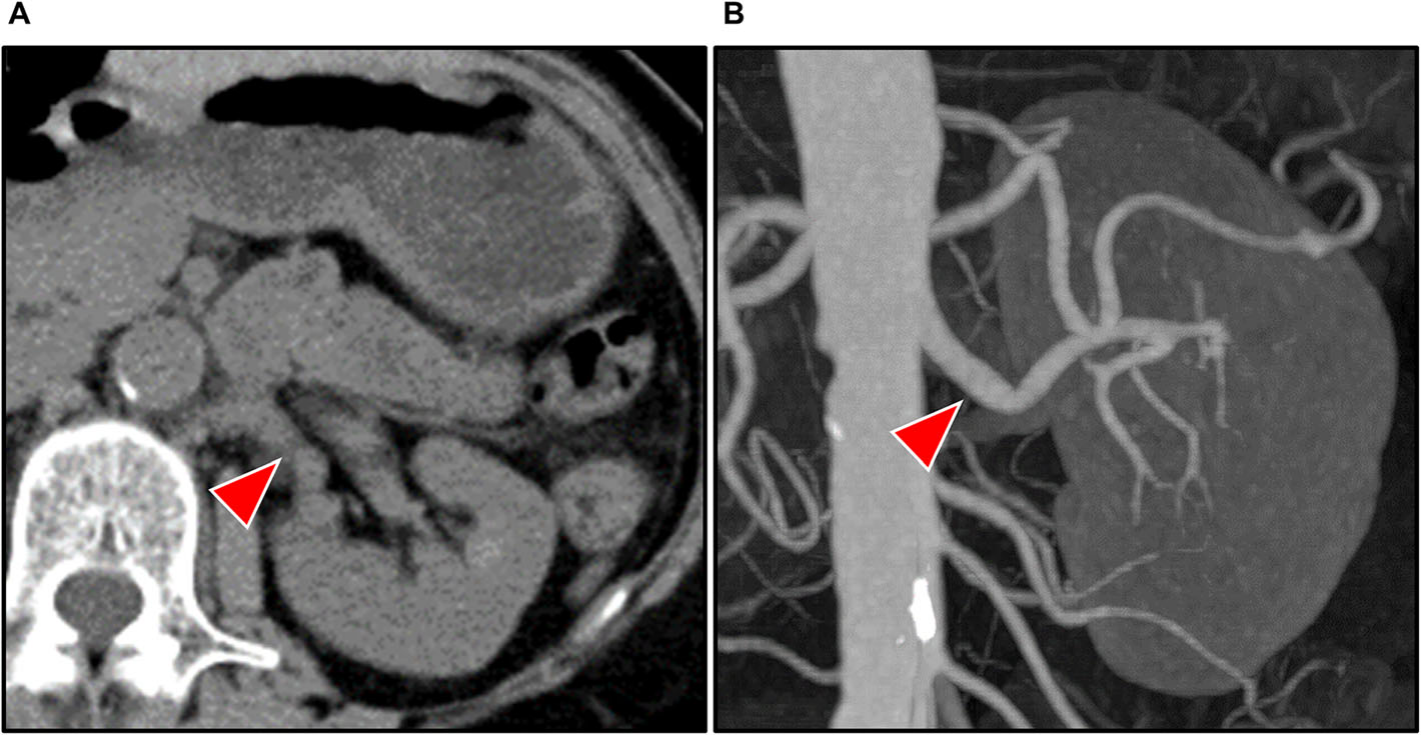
Representative images of the allograft obtained by presurgical unenhanced computed tomography. The donor was the recipient’s mother who was 62 years old, who was good in shape and had no complication. Her left renal artery shows no evidence of arteriosclerosis (red arrow: a axial image; b three-dimensional image)
